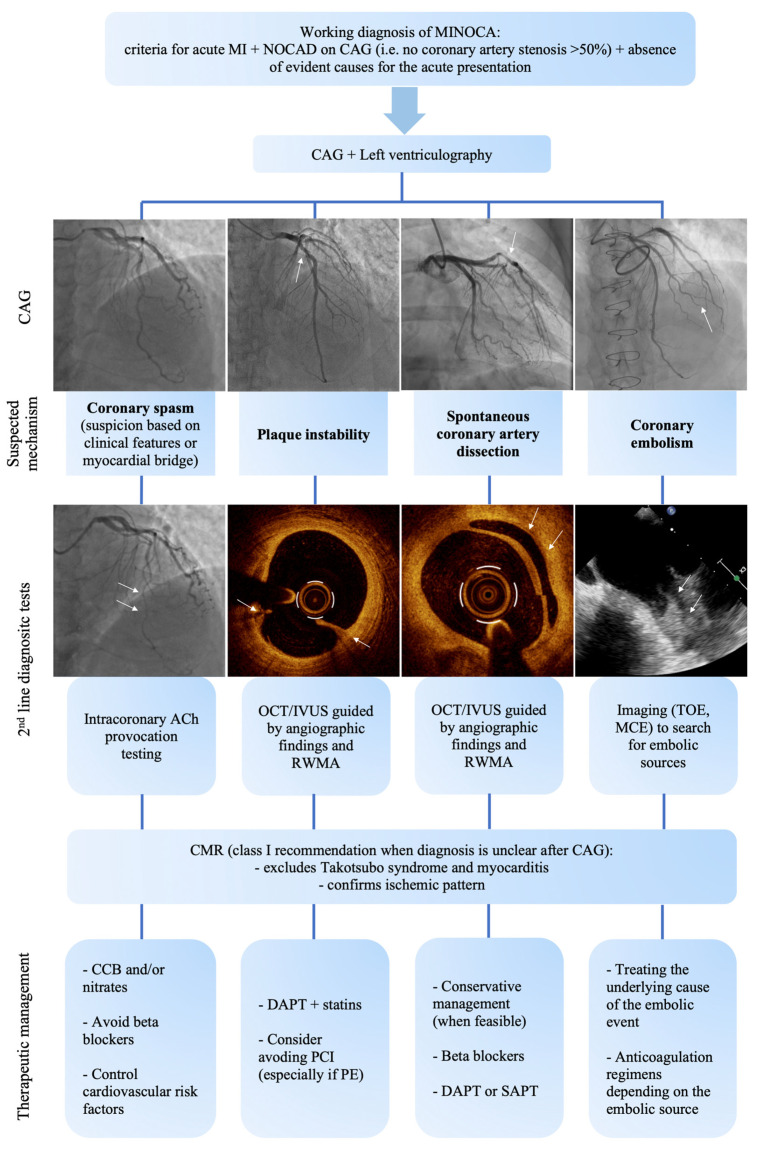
Tailored diagnostic and therapeutic approach in myocardial infarction with non-obstructive coronary arteries. Abbreviations: ACh: acetylcholine; CAG: coronary angiography; CCB: calcium channel blocker; CMR: cardiac magnetic resonance imaging; DAPT: dual antiplatelet therapy; IVUS: intravascular ultrasound; MCE: myocardial contrast echocardiography; MI: myocardial infarction; MINOCA: myocardial infarction with non-obstructive coronary arteries; NOCAD: non-obstructive coronary artery disease; OCT: optical coherence tomography; PE: plaque erosion; RWMA: regional wall-motion abnormalities; SAPT: single antiplatelet therapy; TOE: transesophageal echocardiography.
